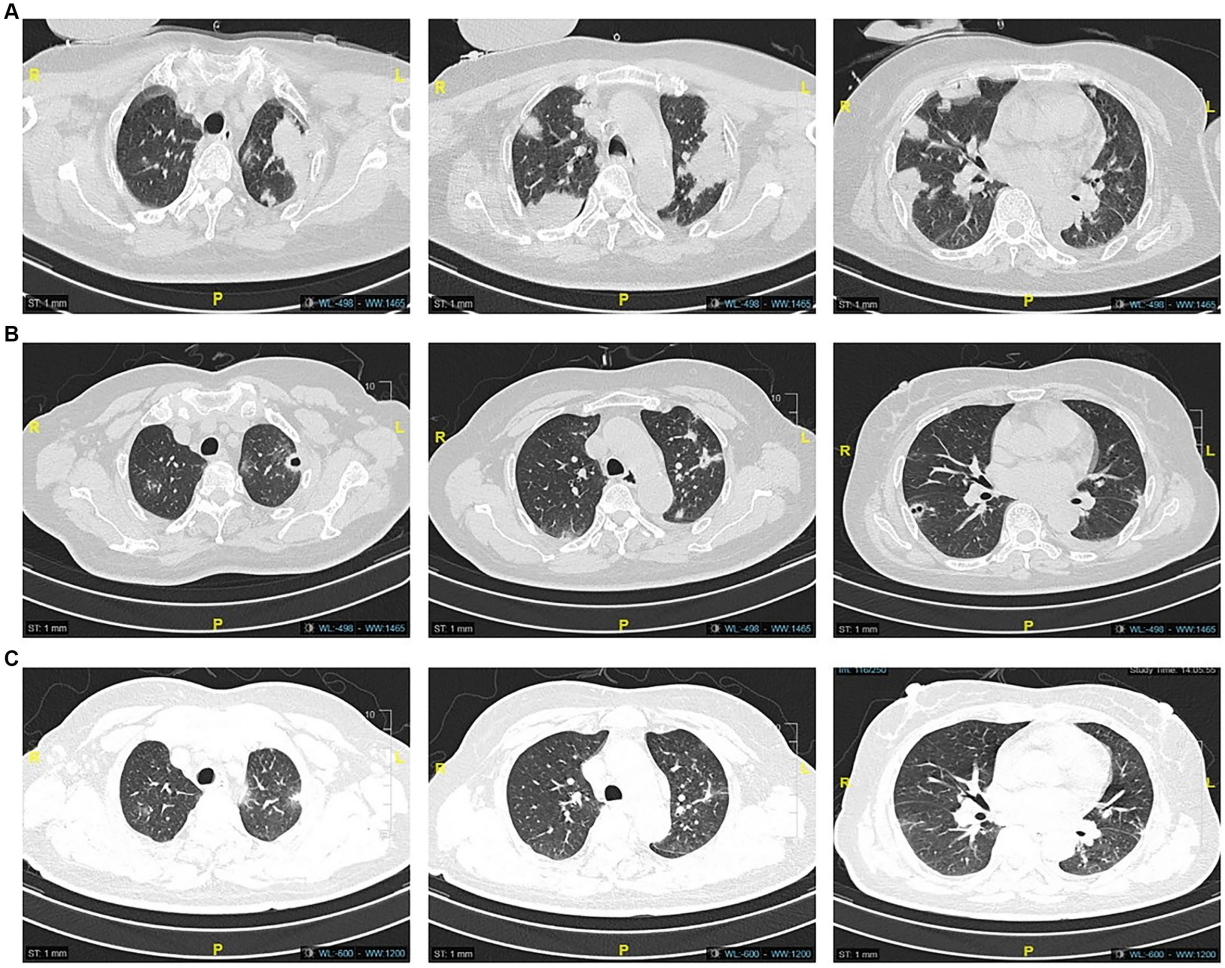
Computed tomography images on admission showed multiple mass shadows (A), and CT images after 15 days of piperacillin-tazobactam anti-infection treatment showed partial resorption of the lung lesions in comparison (B). The lesions continued to resorb after 30 days (C).
